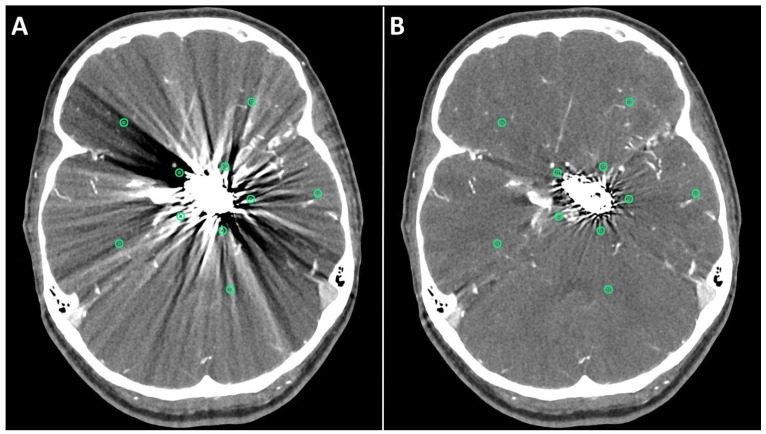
Positioning of ROIs (Region of Interest) for quantitative image analyses on standard (A) and SEMAR (B) CT-angiography images in axial plane (0.25 mm slice thickness) in direct proximity and more distally to the metal implant. In this case, follow up CT-angiography of a 56-year-old female who underwent coil-embolization of a left sided posterior communicating artery aneurysm.
